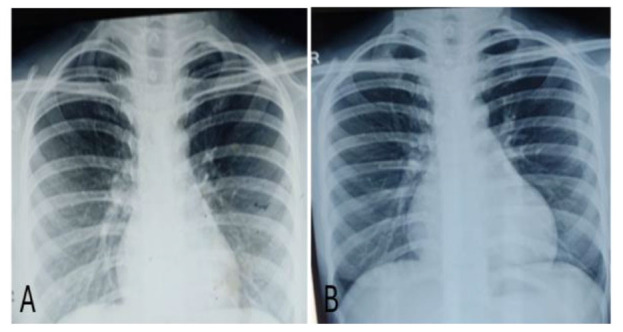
X-ray posteroanterior view before (A) and after (B) starting liposomal amphotericin B therapy
